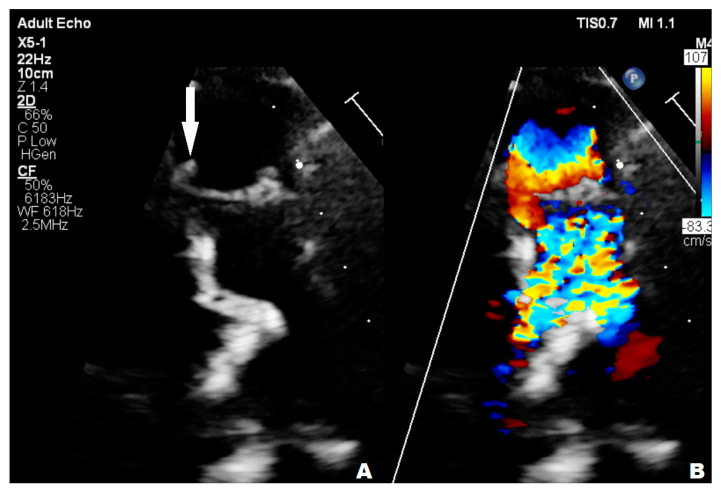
Transthoracic echocardiography; (A) the arrow point at ballotable vegetations; (B) II degree PV regurgitation; PV—pulmonary valve.
